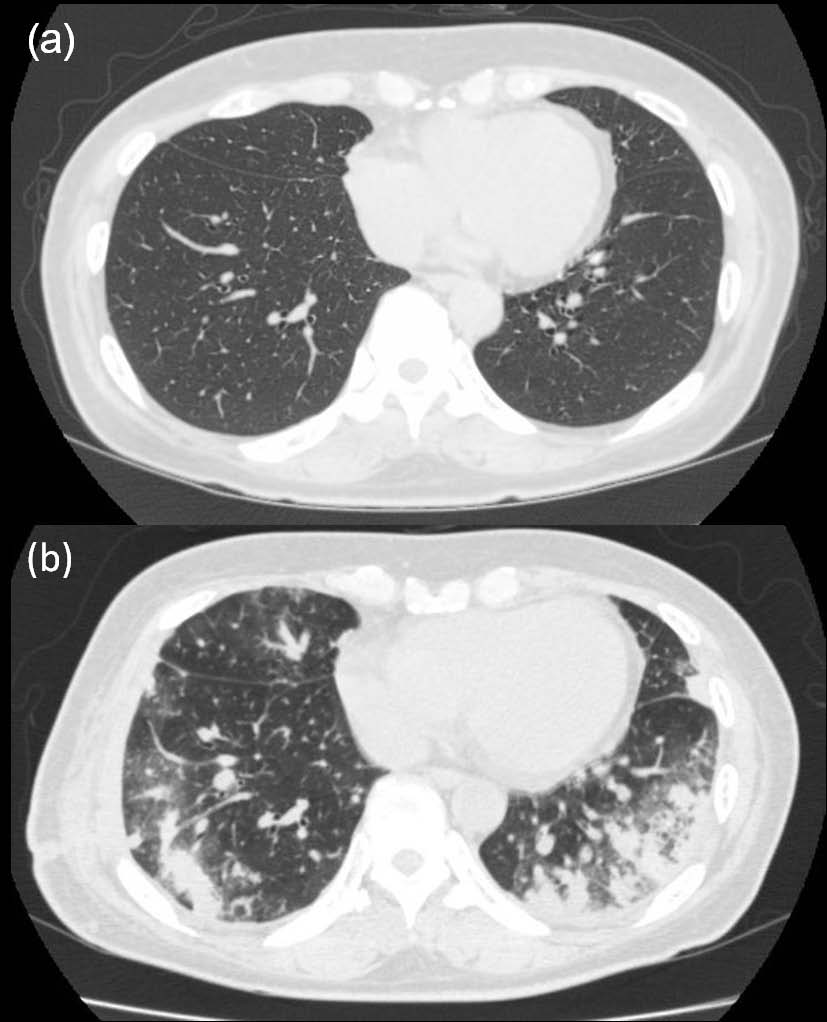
Chest CT scan*. *(a) Preoperative CT scan showing no pulmonary metastasis. (b) Postoperative CT scan showing pulmonary non-segmental consolidation, predominantly in the peripheral lung field, with surrounding ground-glass opacities; no bronchovascular bundle thickness or interlobular septal thickness was observed*. The tumor was resected together with the right seventh, eighth, and ninth ribs.
